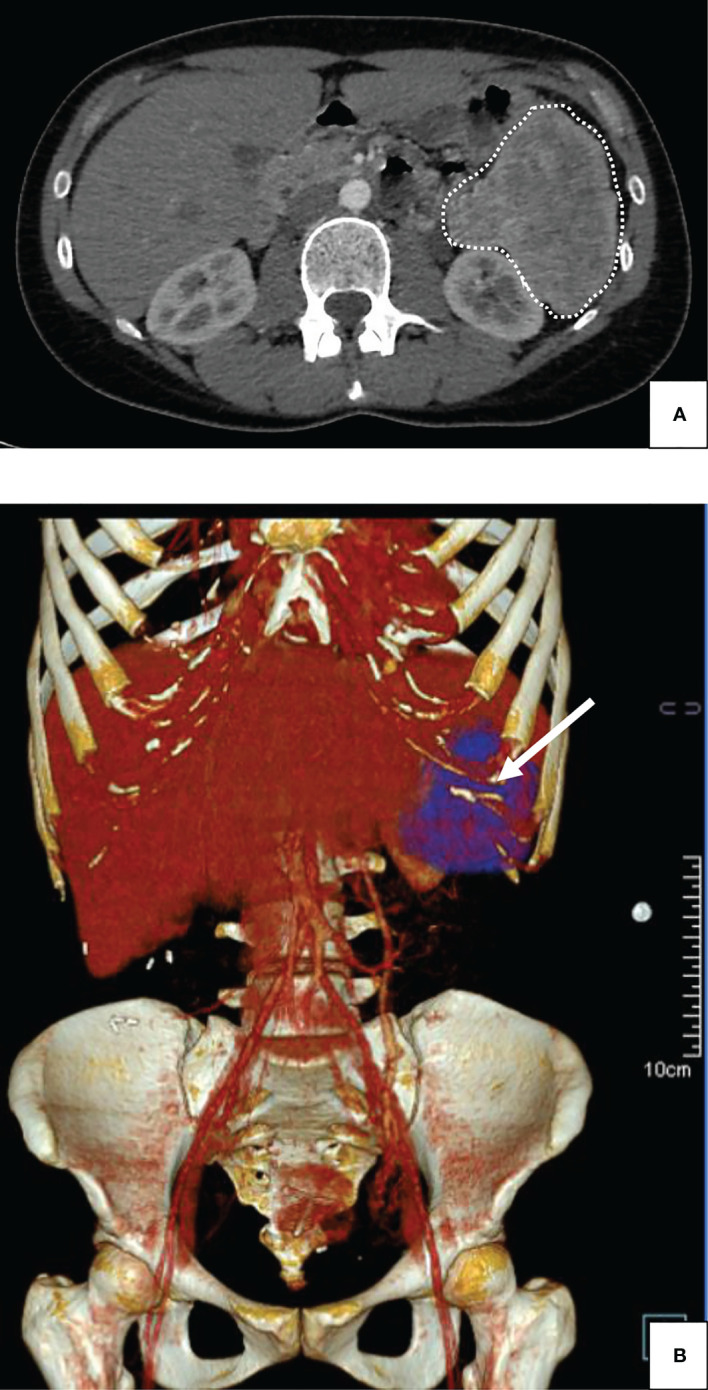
Abdominal injected CT-scan of a giant insulinoma in a 38-year-old female patient suffering from severe hypoglycemia. (A) Plain Computed Tomography scan (CT-scan); horizontal cut: the 88 mm-wide mass was observed in the left hypochondrium, without invasion of adjacent structures (dashed lines). (B) Plain Computed Tomography scan (CT-scan); tridimensional reconstruction: The absence of organ invasion was confirmed using three-dimensional reconstruction for computed tomography (arrow).
